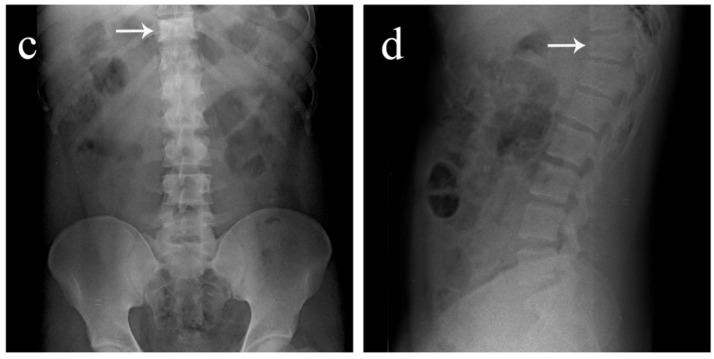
Antero-posterior and lateral view plain radiographs of a 35-year-old male who was brought to the emergency department with multiple abrasions over the trunk and pain in the upper back following a motor vehicle accident. He was conscious and well oriented with stable vitals. Upon examination, midline tenderness was elicited throughout his upper back. His motor power was 5/5 in all four limbs, and there was no sensory deficit. Plain radiographs revealed an obvious anterior wedge compression fracture of the T11 thoracic vertebra. However, since tenderness was elicited throughout the upper thoracic spine and not confined just to the T11 level, more fractures were suspected. On further examination of the anteroposterior view radiograph, it was noticed that the spinous process shadows of the upper thoracic vertebra were either absent or deviated. This brought suspicion of multiple spinous process fractures, which were later confirmed using a computed tomography (CT) scan. (a) Antero-posterior view of the thoracic spine showing absent or deviated spinous process shadows throughout the upper thoracic spine, raising suspicion of spinous process fractures. (b) Lateral view of the thoracic spine showing a compression fracture of T11 vertebrae (arrow). (c) Antero-posterior view of the lumbar spine where the T11 vertebra appears to be sclerotic (arrow). (d) Lateral view of the lumbar spine showing normal lordosis and the T11 compression fracture (arrow).
